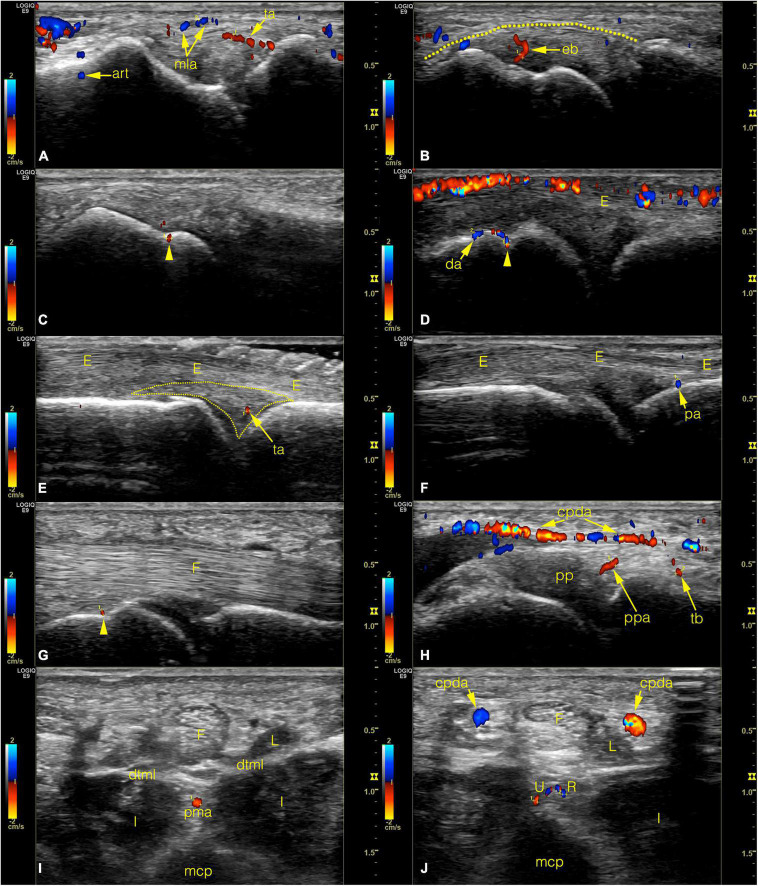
Color Doppler ultrasonographic images of normal joint vessels on healthy volunteers. (Left side of the images refers to the metacarpal half.) (A–C) radial side of the 2nd joint on coronal plane. On panel (B) the dotted line indicates the outer border of the enthesis. (D–F) dorsal side of the joint on sagittal plane images. Note the superficial cutaneous vein along the top of panel (D). On panel (E) the dotted line indicates the dorsal triangle. (G,H) palmar side on sagittal plane. (I,J) axial plane images with the homuncule shaped soft tissue complex in the center with palmar metacarpal artery and it’s bifurcation into R- and U-branches, respectively. arrowhead, entry point of a bone supplying vessel; art, Doppler mirror artifact; cpda, common palmar digital artery; da, dorsal arcade; dtml, deep transverse metacarpal ligament; eb, enthesial branch; E, extensor tendon; F, flexor tendon; I, interosseous muscle; L, lumbrical muscle; mcp, metacarpal; mla, main lateral artery; pa, phalangeal arcade; pma, palmar metacarpal artery; pp, palmar plate; ppa, palmar plate artery; ppda, proper palmar digital artery; R, R-branch; ta, triangular arcade; tb, tenosynovial trunk; U, U-branch.
